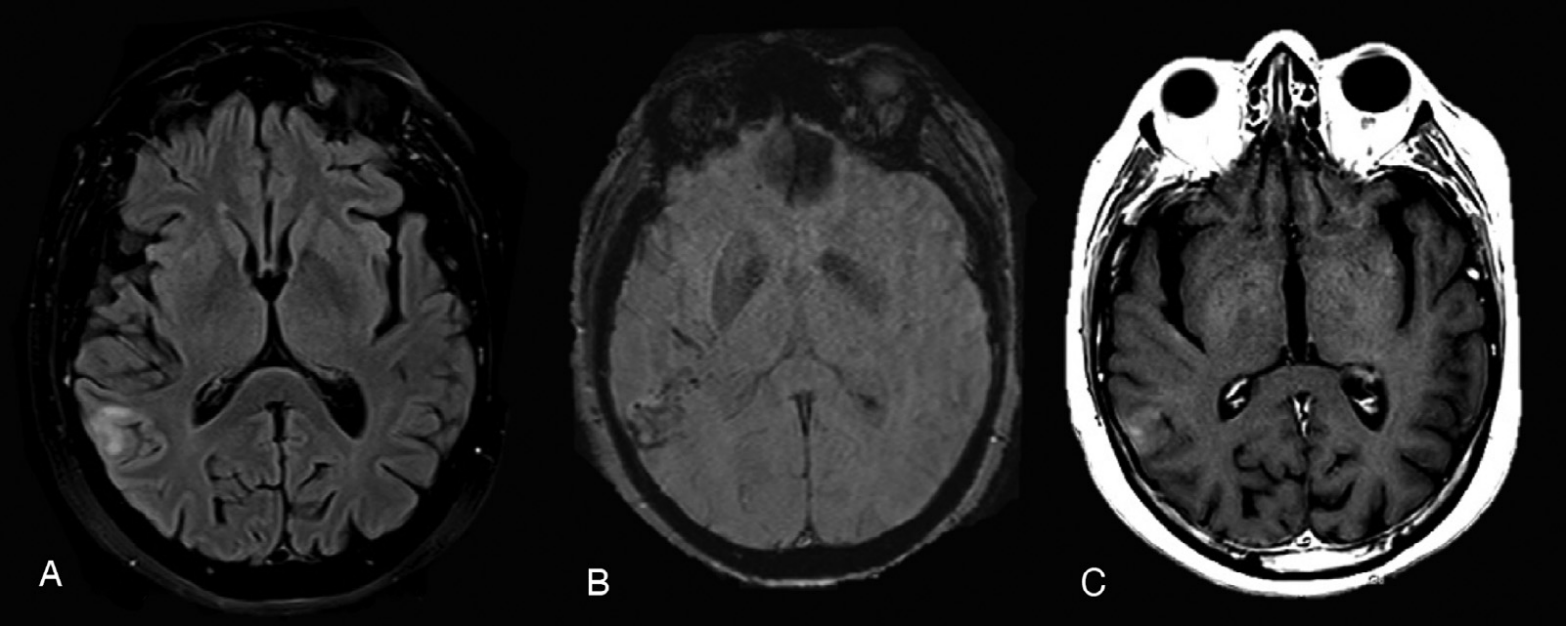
(A) FLAIR ax; (B) SWI ax; post contrast T1 SE ax. Two months later a follow-up MRI study showed the onset of a subcortical area of white matter hyperintensity on FLAIR images (A) in the right parieto-temporal region arising on previous haemorragic foci (B). After contrast administration, the exam showed blurred contrast-enhancement in the same involved WM area (C).
